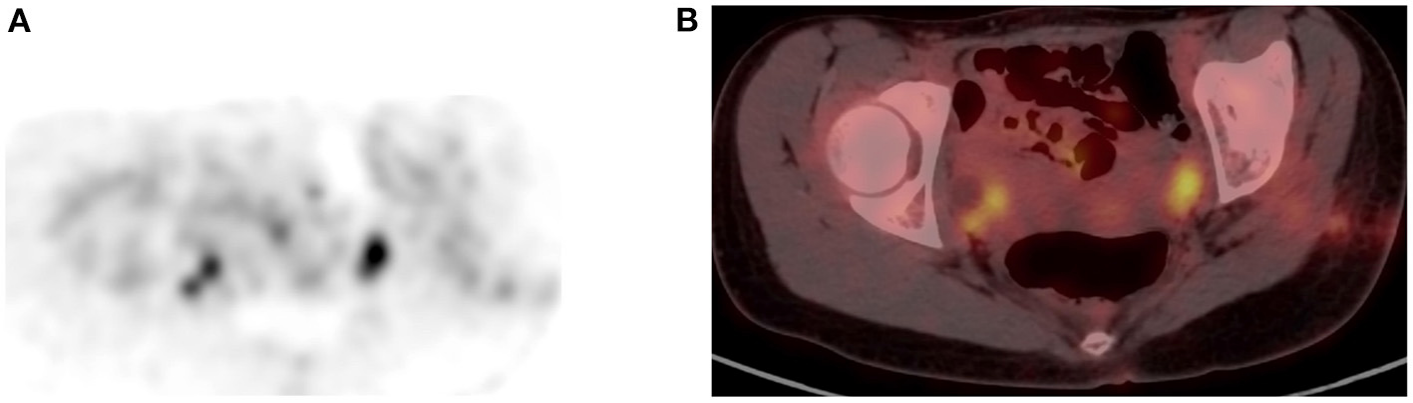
Uptake in ovaries. FDG PET Axial (A) image showed focal increased uptake in pelvis corresponding to ovaries in combined PET/CT (B) image, in a female patient of childbearing age. The uptake is physiologic due to menstrual cycle.
